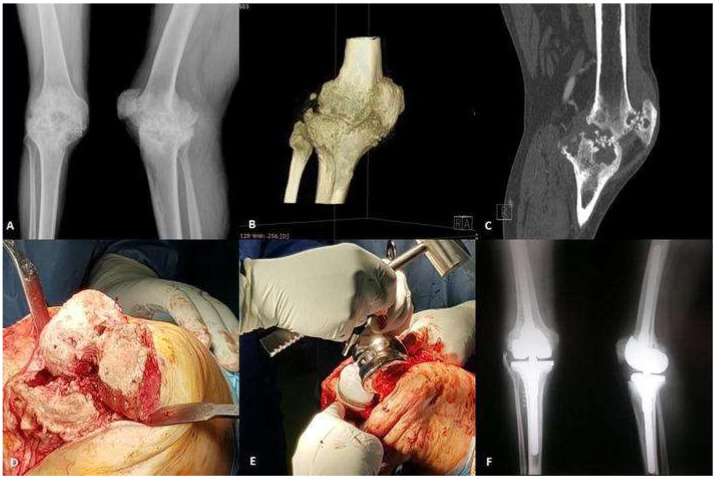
Total knee replacement in a haemophilic patient receiving concentrate replacement therapy. (A) Severe right gonarthrosis with bilateral genu valgum, more visible on the right side. (B) Typical shape and structure modifications for a haemophilic knee arthropathy (widening of the tibial plateau, tibial condyle hypertrophy, accentuation of the intercondylar incision with modification of the tibial spines and obvious rarefaction of the geodesic structure. slight collapse of the right internal tibial plateau). (C) periarticular osteolytic lesions with interruption of the cortex at the level of the tibial plateau and the condyles of the femur and at the level of the posterior patellar surface, with sclerotic edges and subcortical cysts.; narrowing of the femoral-tibial joint space until complete disappearance (D) and (E). Total knee arthroplasty for severe haemophilic arthropathy using a revision modular prothesis “rotating-hinge” type-intraoperative images. (F) Right knee total arthroplasty-intraoperatively front and lateral radiography.
